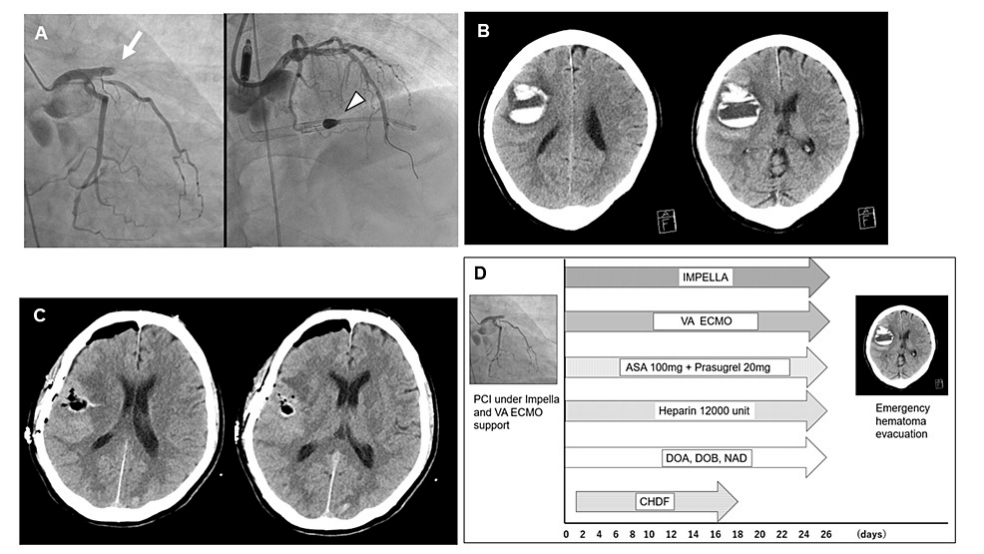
Clinical course of case two A) Images of left anterior descending artery before (left) and after (right) percutaneous coronary intervention:  Complete occlusion of left anterior descending artery was treated by percutaneous transluminal angioplasty and stent placement. Impella was implanted to treat cardiogenic shock; B) Intracerebral hemorrhage in the left frontal and temporal lobes: The hematoma volume is 59.3 mL; C) Computed tomography after emergency hematoma evacuation shows the removal of hematoma; D) Graph showing the treatment process of case two from admission to onset of intracerebral hemorrhage. VA ECMO - venoarterial extracorporeal membrane oxygenation; PCI - percutaneous coronary intervention; ASA - acetylsalicylic acid
